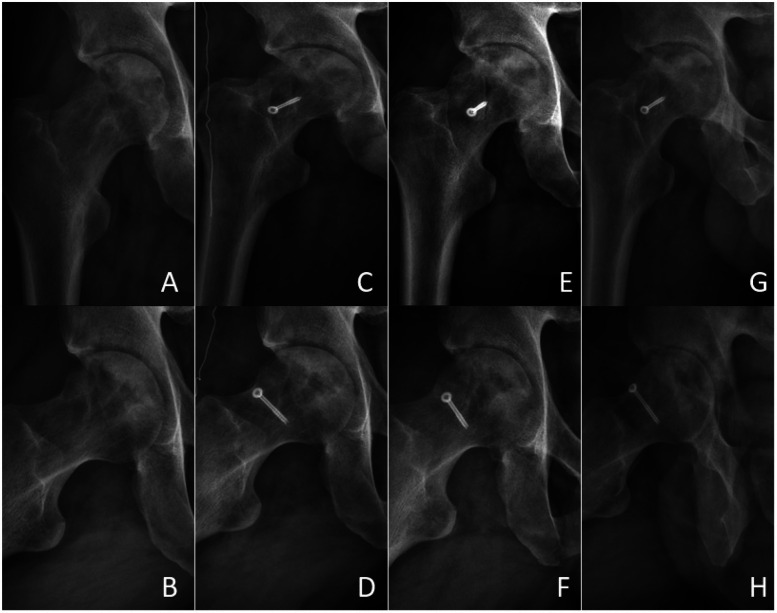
Anteroposterior and frog-leg x-ray images of the right hip joint in a typical patient. Preoperative anteroposterior and frog-leg films (A,B); 1 day postoperative anteroposterior and frog-leg films (C,D); 3 months postoperative anteroposterior and frog-leg films (E,F); 6 months postoperative anteroposterior and frog-leg films (G,H).
